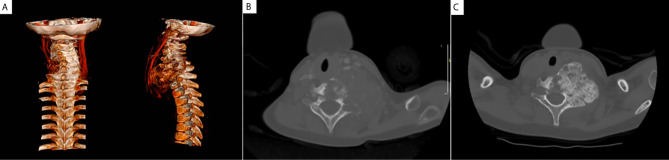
Aneurysmal bone cyst at diagnosis. MRI with 3D reconstruction at diagnosis (A) showing the calcified lytic lesion originating from C4-C7 hemivertebrae and encaging the vertebral artery, preventing invasive surgery; CT at diagnosis (B) identified a bulky lesion originating from C4-C7 hemivertebrae. CT performed 6 months after the introduction of denosumab (C) showed a massive calcification of the lesion that remained stable in size.
